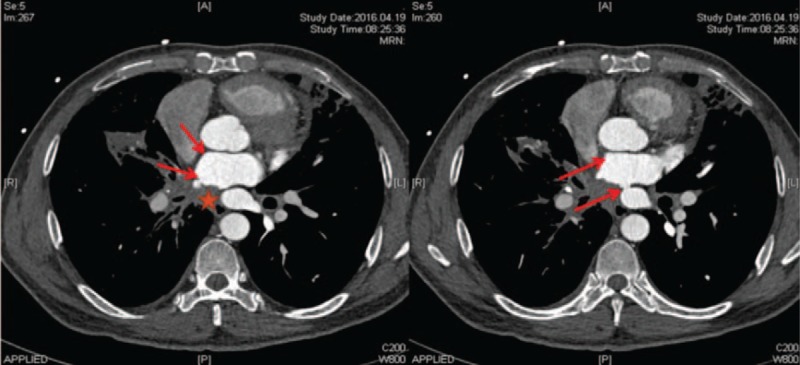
Chest CT (mediastinal window) in patient with FM. Multiple soft-tissue shadows (asterisk) are evident. Left and right superior PV occlusion and inferior PV stenosis were observed (arrows).
